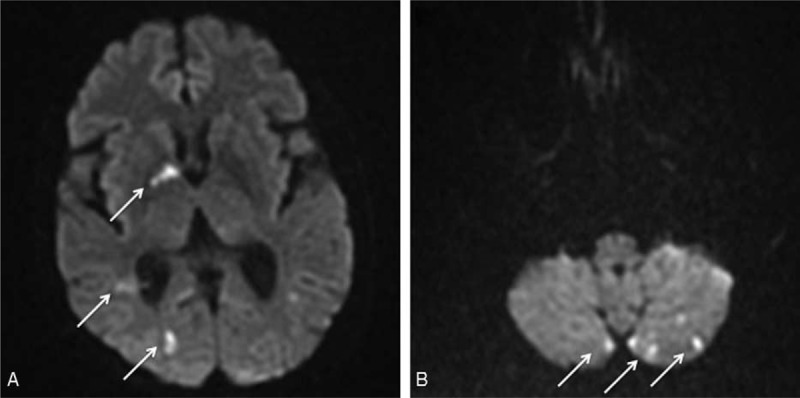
Diffusion-weighted images of cranial MRI. Acute hemispheric (A) and cerebellar (B) ischemic lesions.
